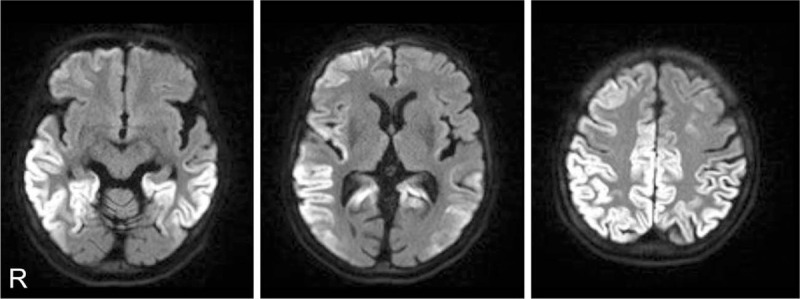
Diffusion-weighted magnetic resonance images obtained at 4 months after symptom onset. Extensive hyperintensities in the bilateral frontal, temporal and parietal cortices are seen.
